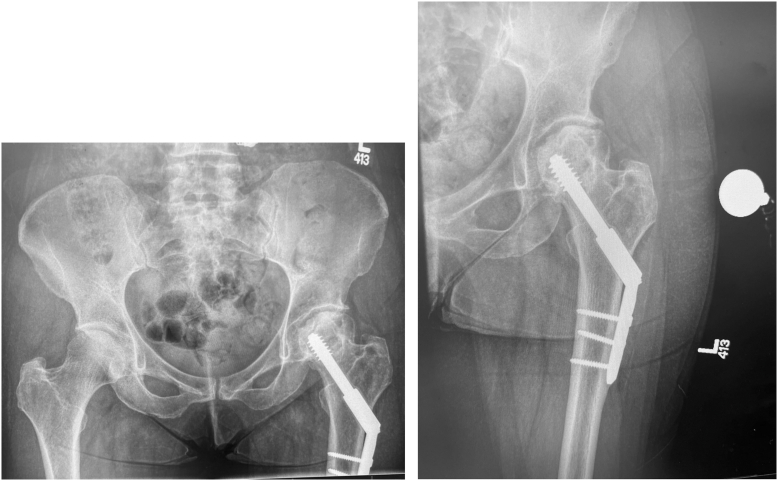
Preoperative anteroposterior pelvis and anteroposterior hip radiographs.
